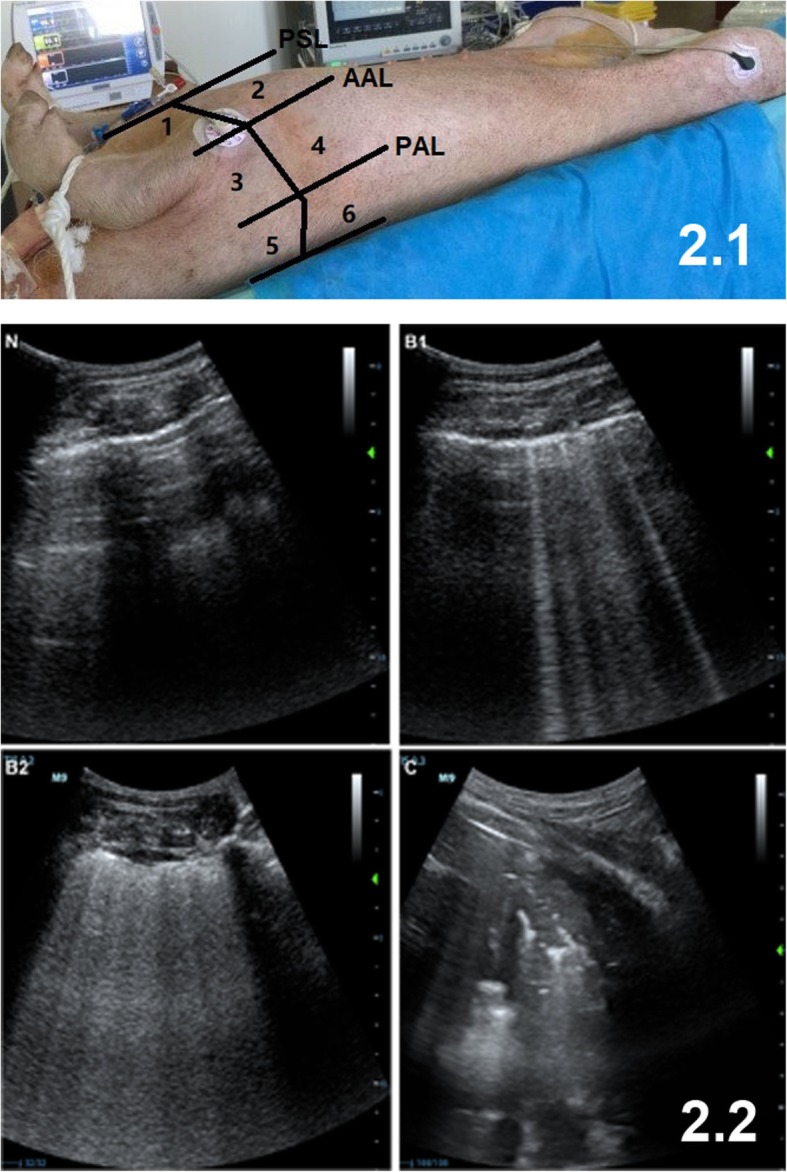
Lung ultrasound scanning. 2.1. Twelve regions of the left and right chest wall in lung ultrasound scanning. PSL, parasternal line; AAL, anterior axillary line; PAL, posterior axillary line. The upper and lower portions are bounded by the midpoint of the sternum; 2.2. Lung ultrasound pattern. N normal (zero); B1 moderate (one point); B2 severe (two points); and C lung consolidation (three points)
